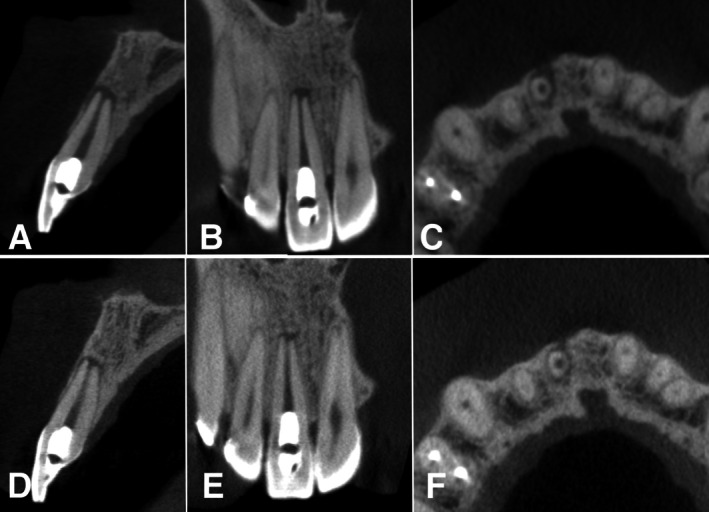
CBTC sagittal (A), coronal (B), and axial (C) images after one year of the endodontic regeneration procedure. Partial formation of the buccal bone plate in the sagittal cross‐section (A), reduction in the periradicular lesion in the coronal and axial cross‐sections (B and C). After 4 years, the new CBCT images show complete formation of the buccal bone plate (D); both the coronal (E) and axial (F) cross‐sections show almost complete healing of the apical lesion.
